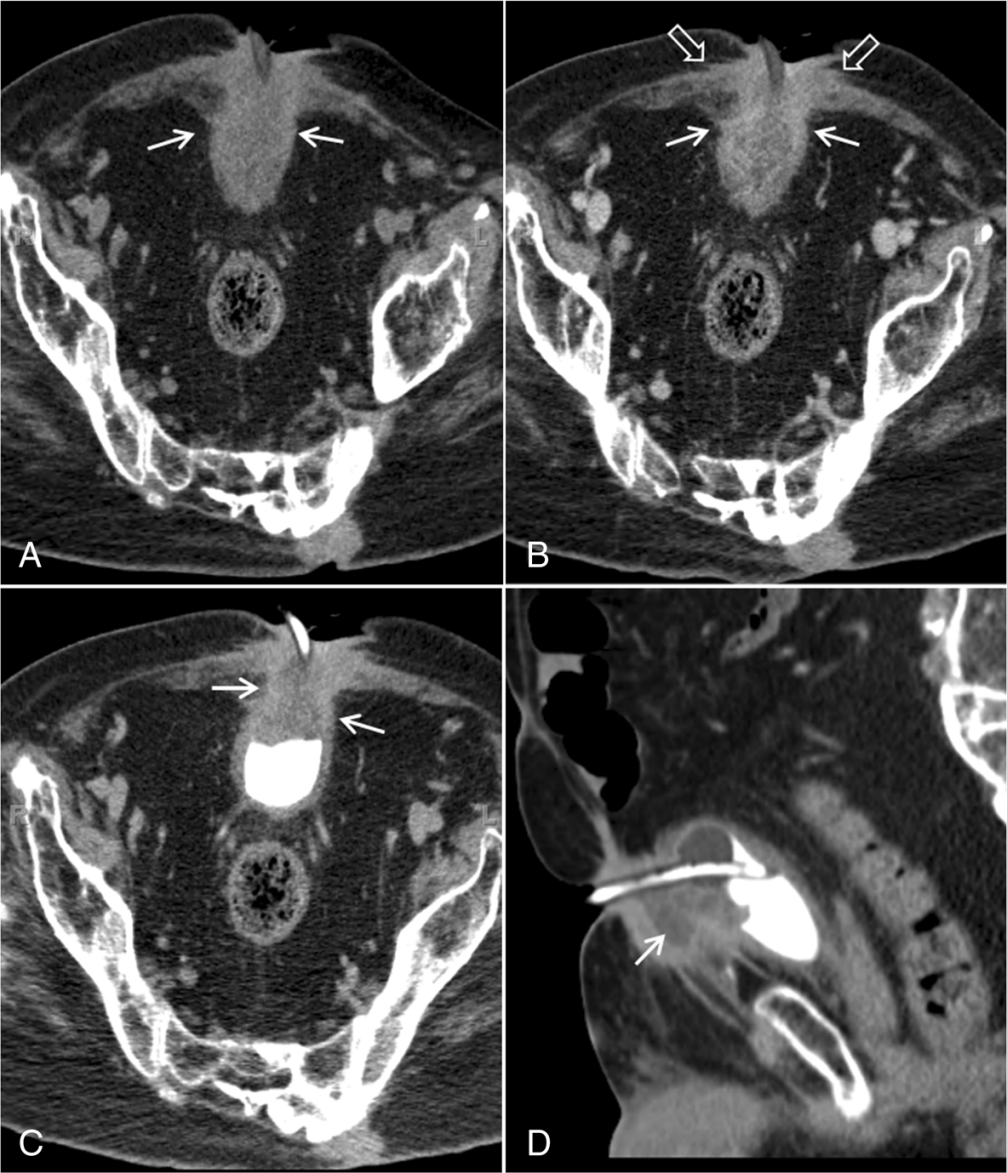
Pre- and post-contrast axial images from CT urography (a and b) as well as axial and sagittal images acquired during the delayed excretory phase (c and d) demonstrate an irregular enhancing soft tissue mass arising along the anterior bladder at the level of the suprapubic catheter and extending along the catheter tract to the skin surface (arrows in a–c). There is infiltration of the anterior abdominal wall (open arrows in b). A low density component of the mass (arrow in d) likely represents intralesional mucin
